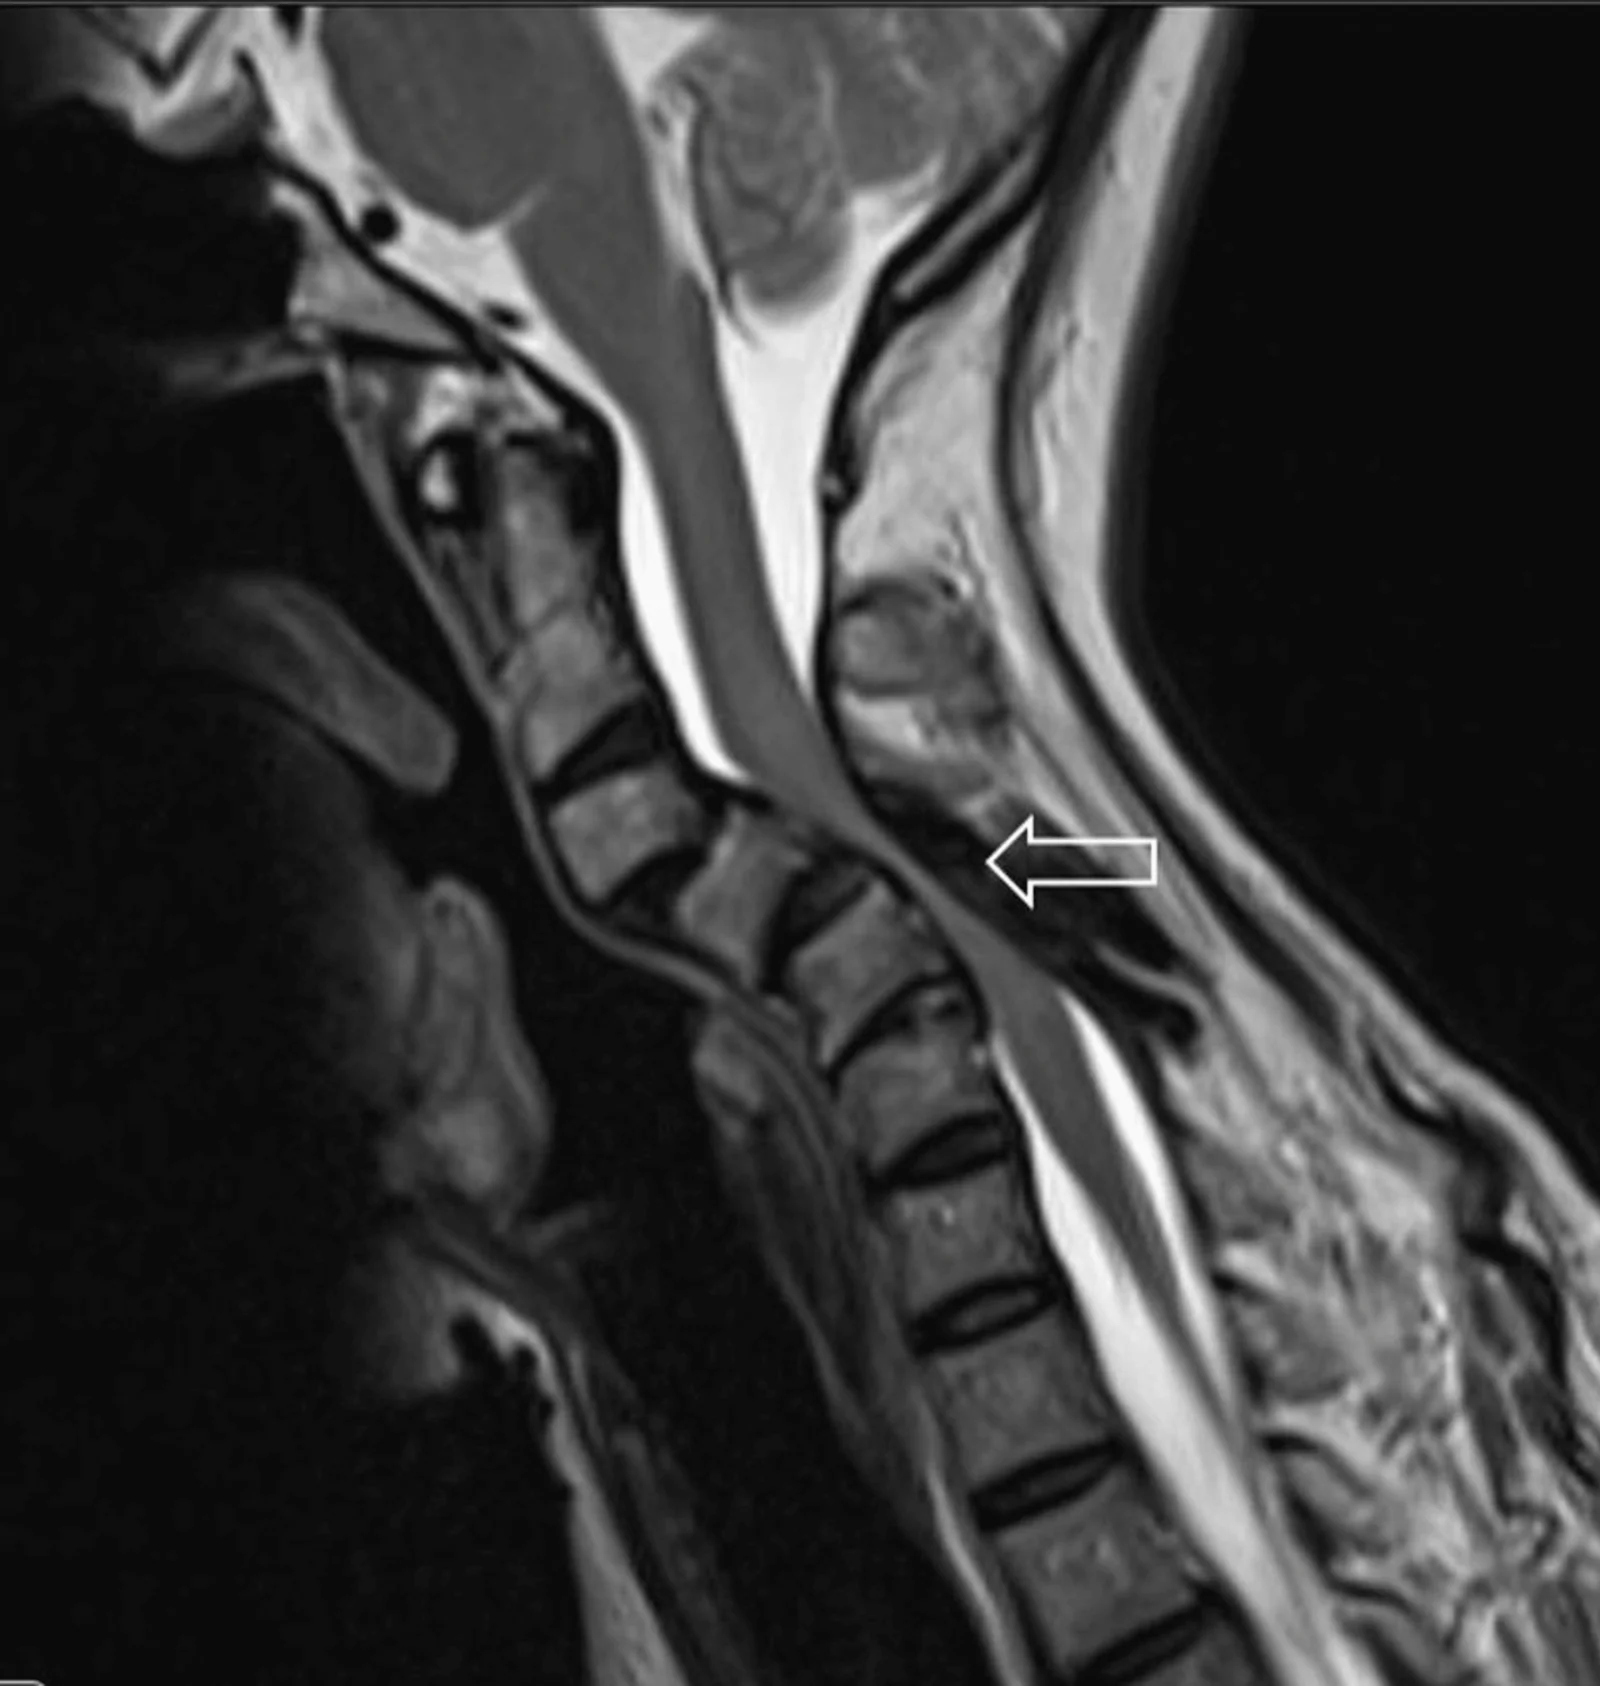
Cervical spine MRI demonstrating severe cord compression with myelomalacia at the C3-4 to C5-6 levels (arrow).
